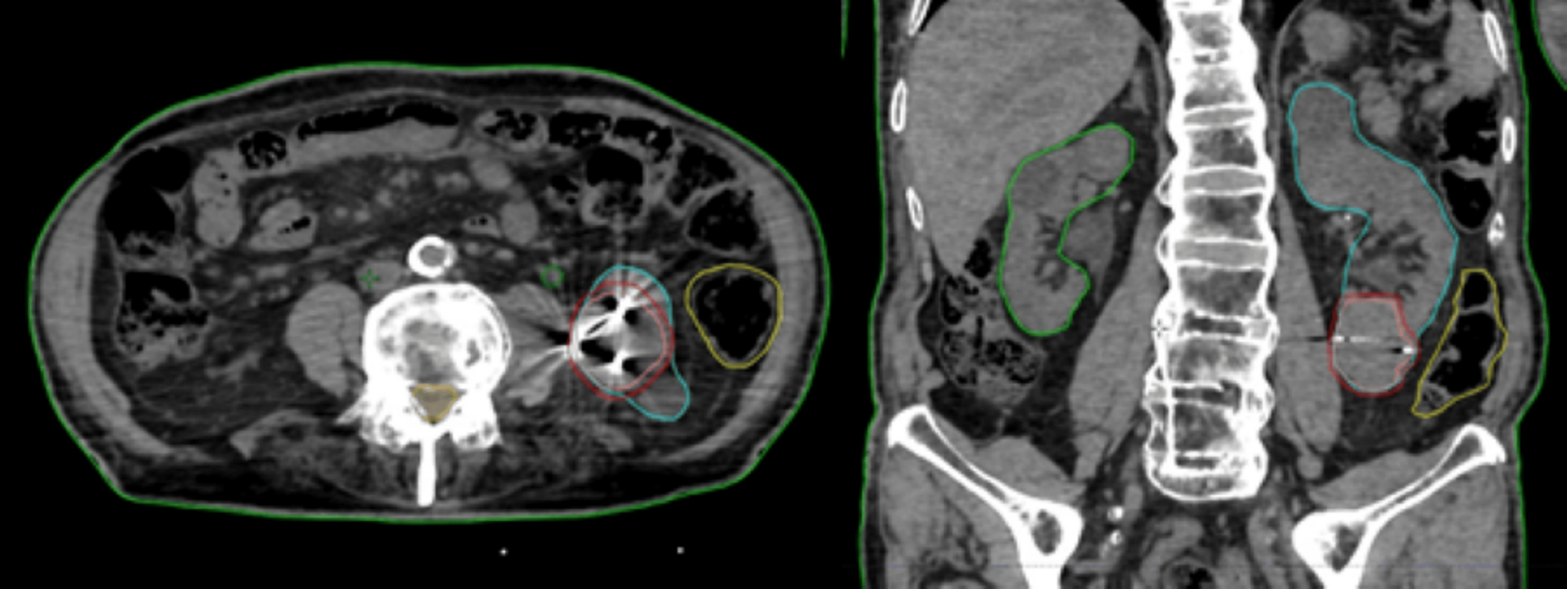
Left renal volume delineation from the treatment planning CT scan in axial (left) and coronal (right) planes
